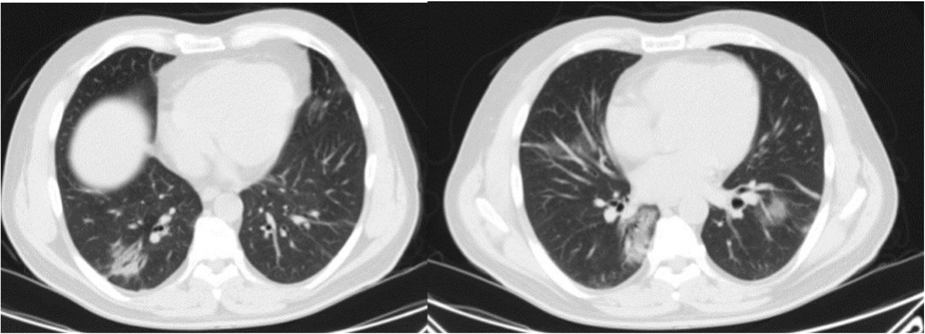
COVID-19 pneumonia: thin section CT shows mass-like peribronchovascular and subpleural consolidation in lower lobes
